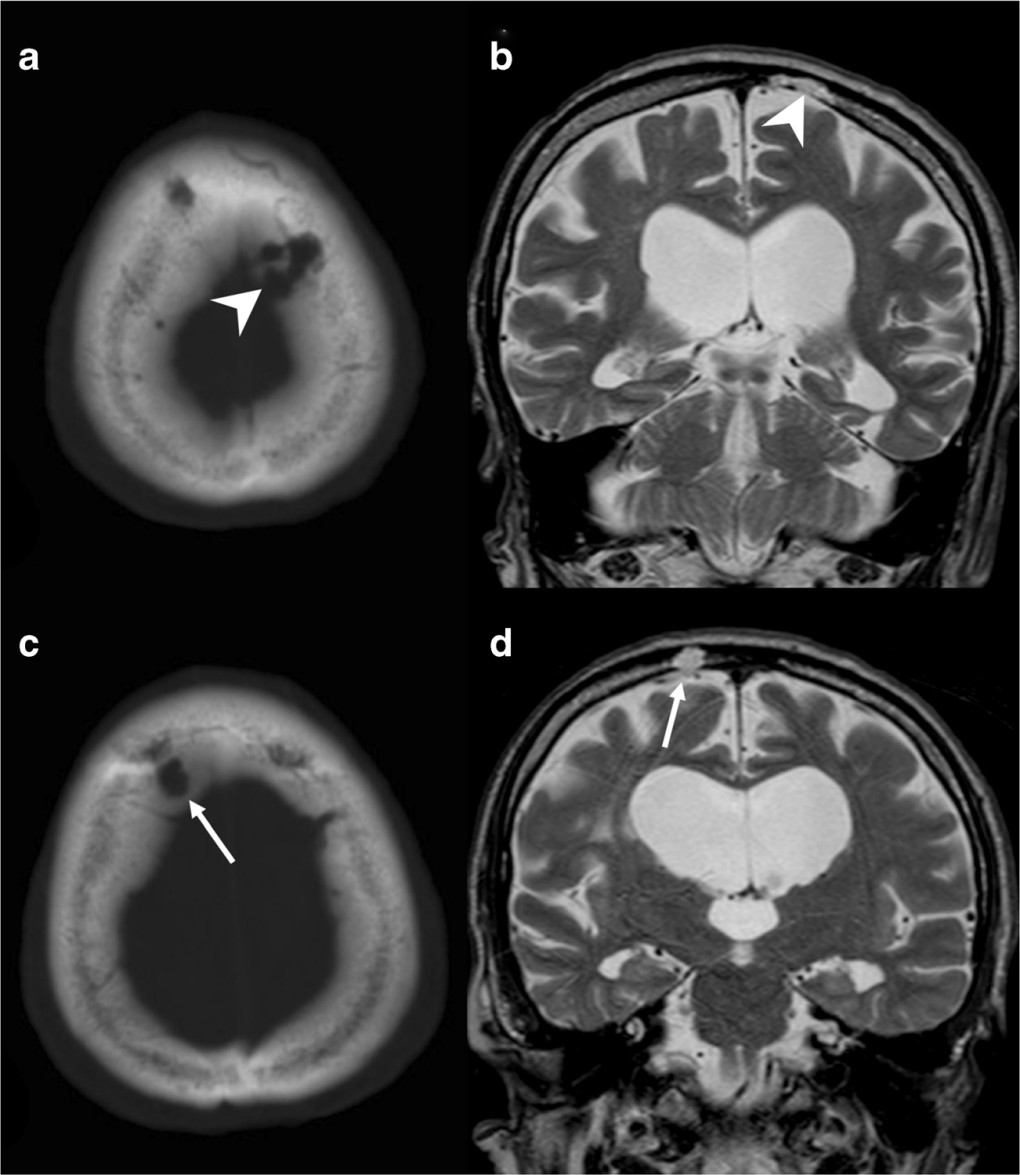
Venous lacuna (VL). Axial CT image with bone (a) window showing a lytic lesion within the left parasagittal diploic space (*arrowhead*), confirmed on T2-weighted sequence (b). In a caudal slice, a right parasagittal AG can be depicted (c, d)
